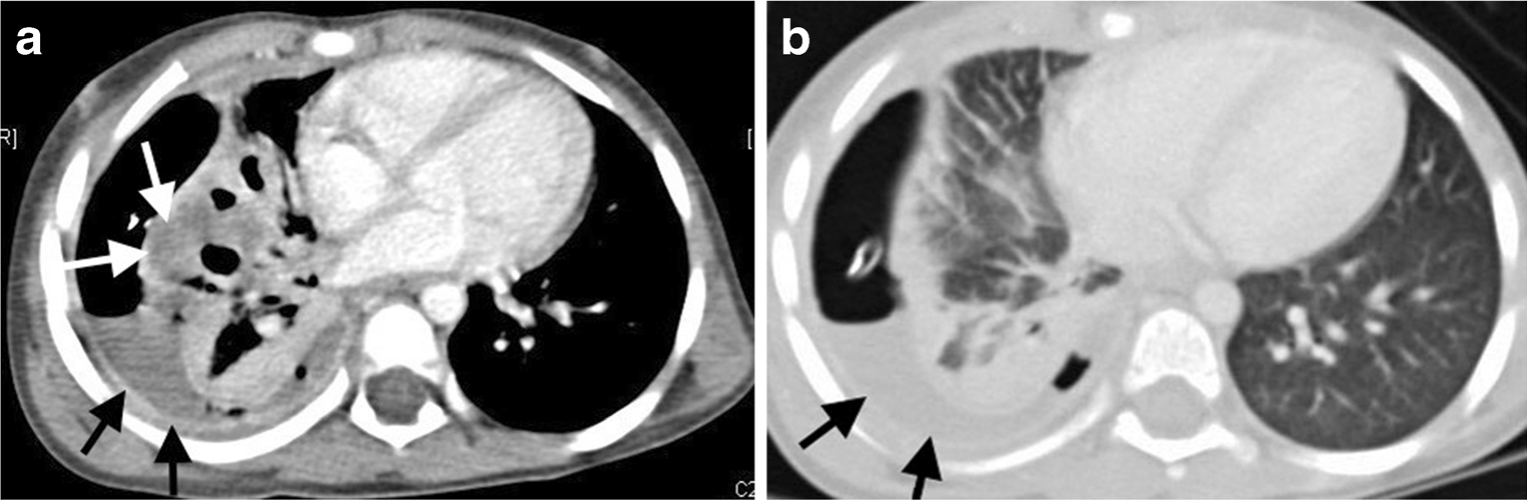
A 6-year old girl with empyema, who has an indwelling right-side chest drain. Contrast-enhanced axial CT of the chest in soft-tissue window (a) and lung window (b) demonstrate that the lung underlying the empyema (*black arrows*) has patchy areas of non-enhancement/low density (*white arrows* in a) indicating necrotising pneumonia without cavitation
